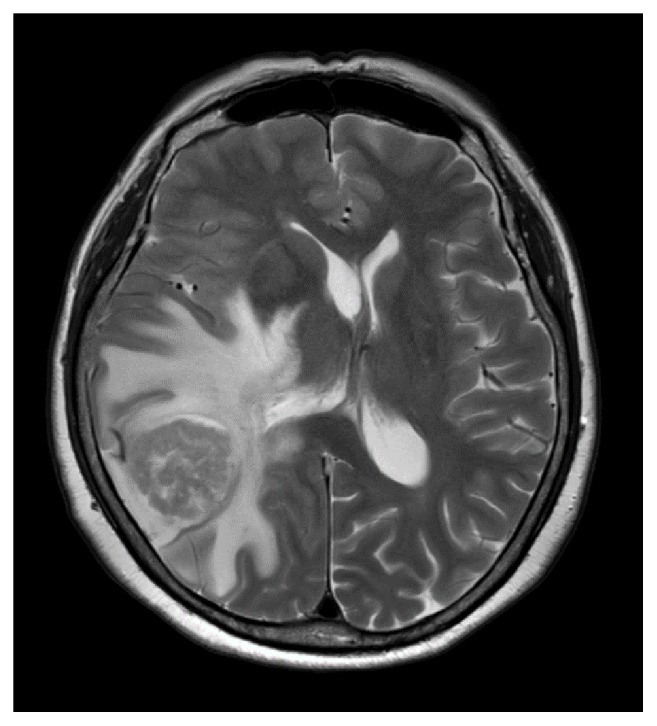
Axial T2-weighted brain MRI scan shows a solitary metastatic lesion in the right temporal lobe with brain edema.
